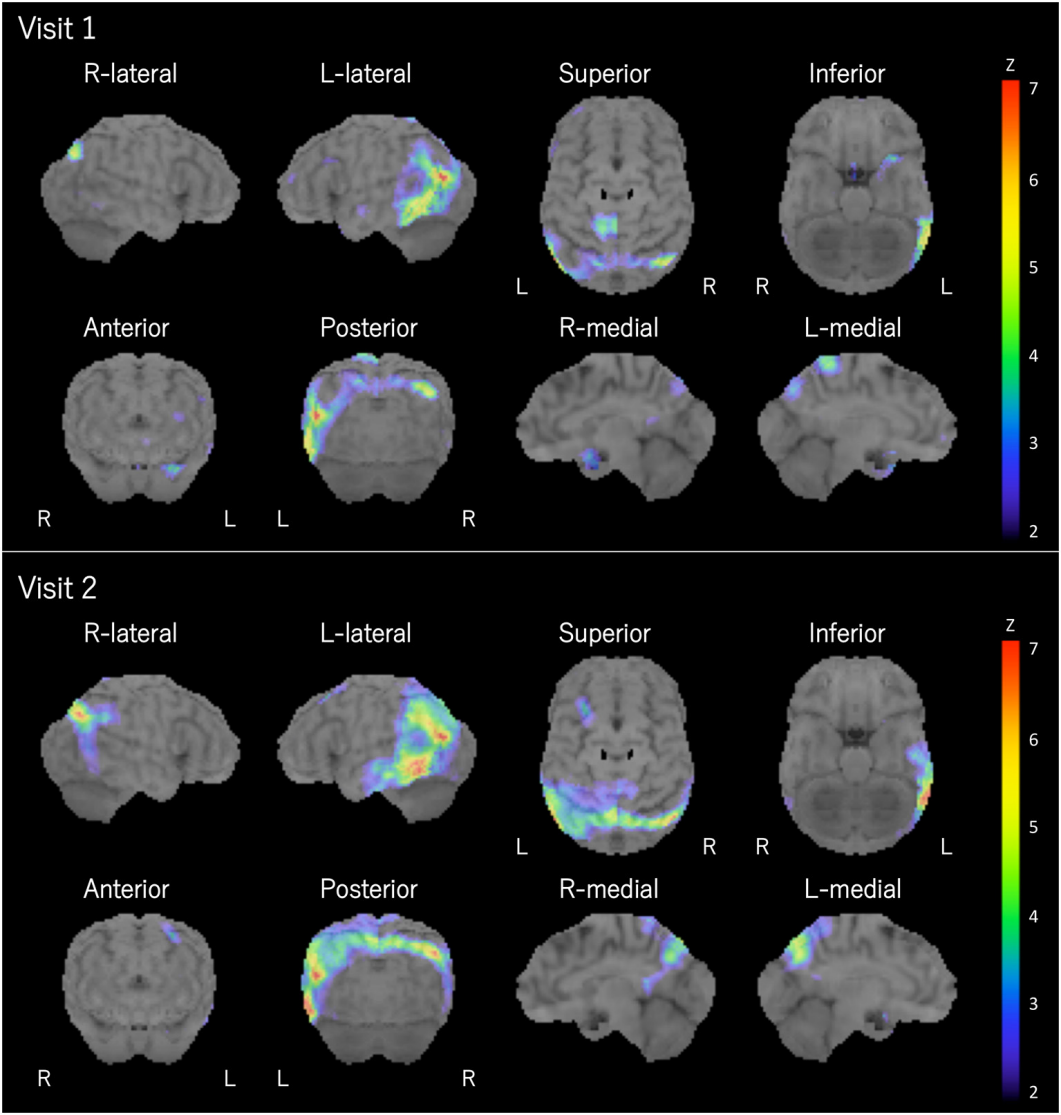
Brain SPECT. Brain SPECT analyzed with an easy *Z*-score imaging system at visits 1 and 2 revealed predominant left-sided hypoperfusion in the temporoparietal lobe. L, left; R, right.
